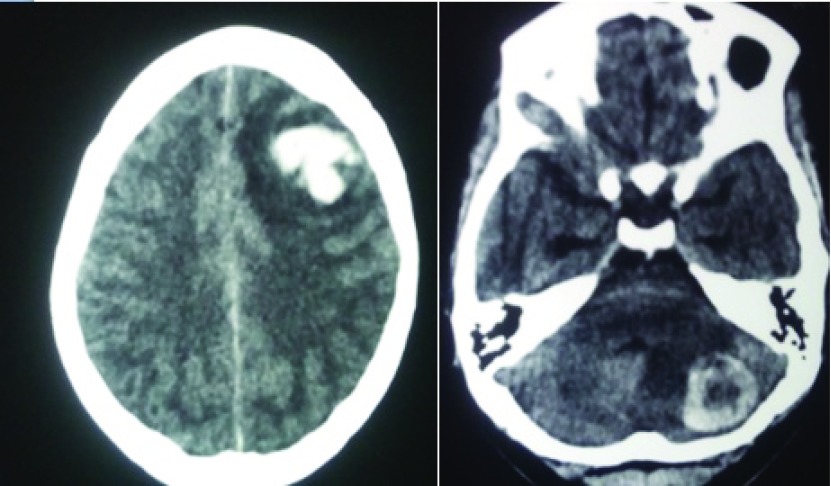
CT head images showing multiple hemorrhagic lesions within the brain.
